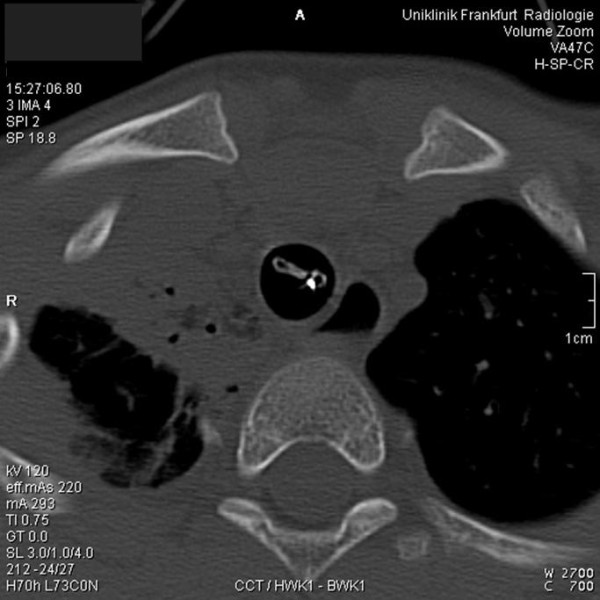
Computed tomography. Transversal plane at the level of the first thoracic vertebra. The endotracheal tube is critically obstructed by the inflated cuff. The radiopaque label (white dot on the scan) indicates the obstructed lumen of the tracheal tube.
